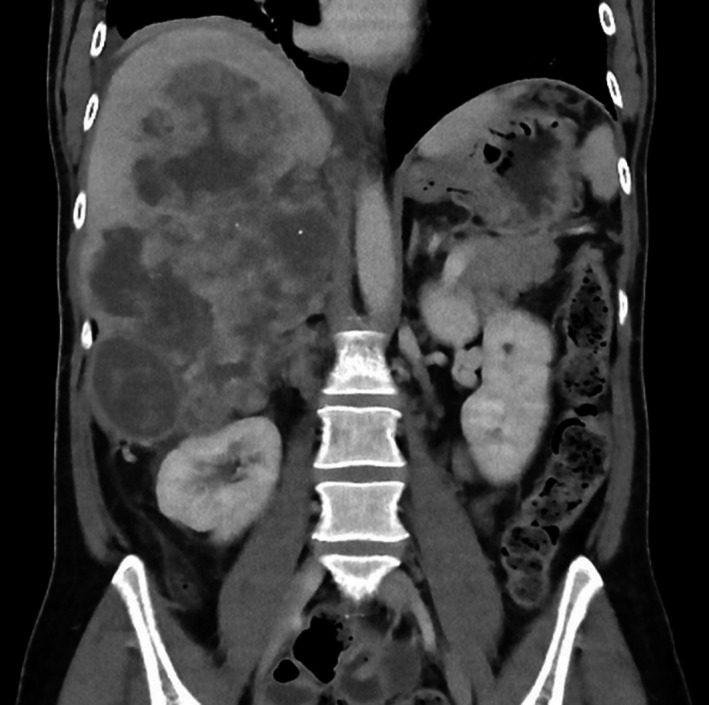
Abdominal CT scan. An axial contrast‐enhanced computed tomography (CT) image of the abdomen shows an inhomogeneous, large, nonenhanced hypodense lesion measuring 13.6 × 11.6 × 20 cm, occupying most of the right liver with exophytic components encroaching the upper right suprarenal region and displacing the right kidney inferiorly
